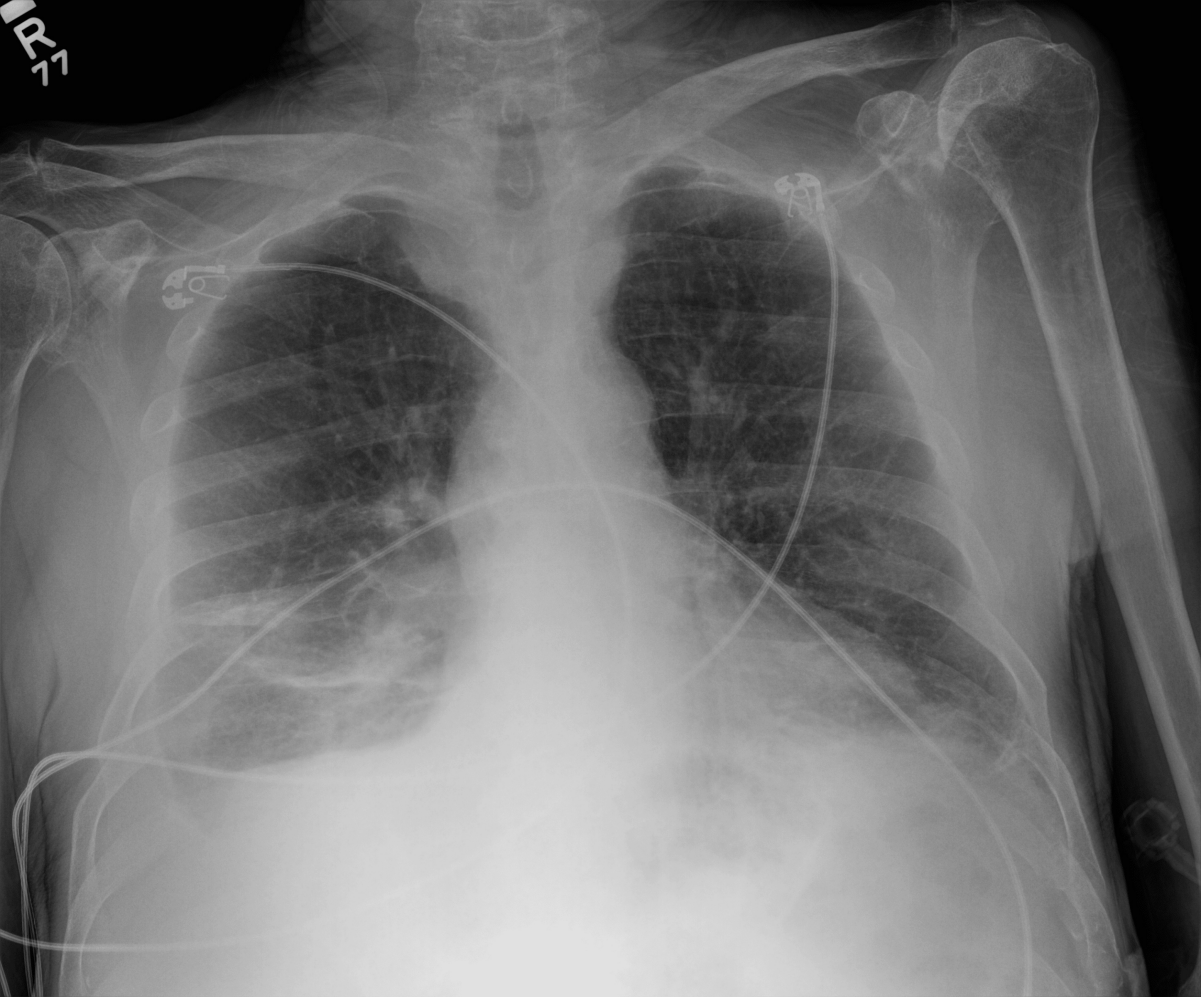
Chest x-ray showing increased opacity in the right lower lobe
